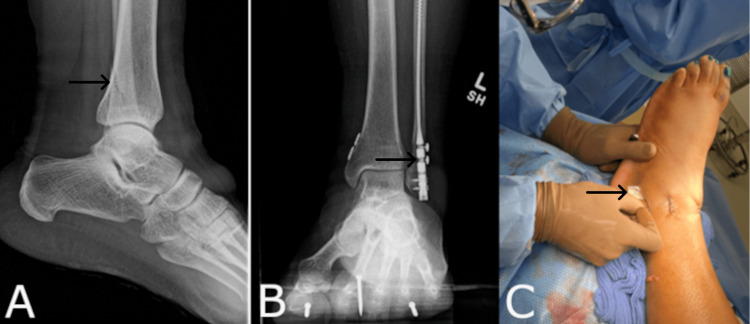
(A) Radiographic evidence of a bimalleolar ankle fracture. (B) Radiographic image of the ankle fracture after undergoing an open reduction internal fixation procedure. (C) The synthetic hybrid-scale fiber matrix being applied to the wound bed during surgical repair of the bimalleolar ankle fracture.
